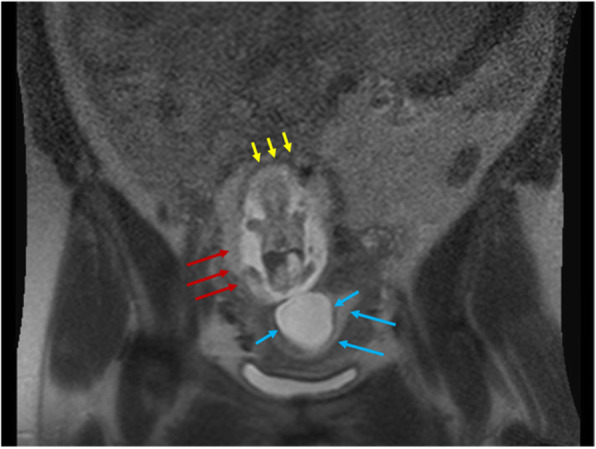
MRI demonstrating the gestational sac enveloped in myometrium (red arrows). The amniotic sac is hour glassing into the endometrial cavity and uterine decidua appears to be located adjacent to gestational sac (blue arrows). A thin layer of myometrium seems to be intact and surrounding the interstitial pregnancy (yellow arrows)
